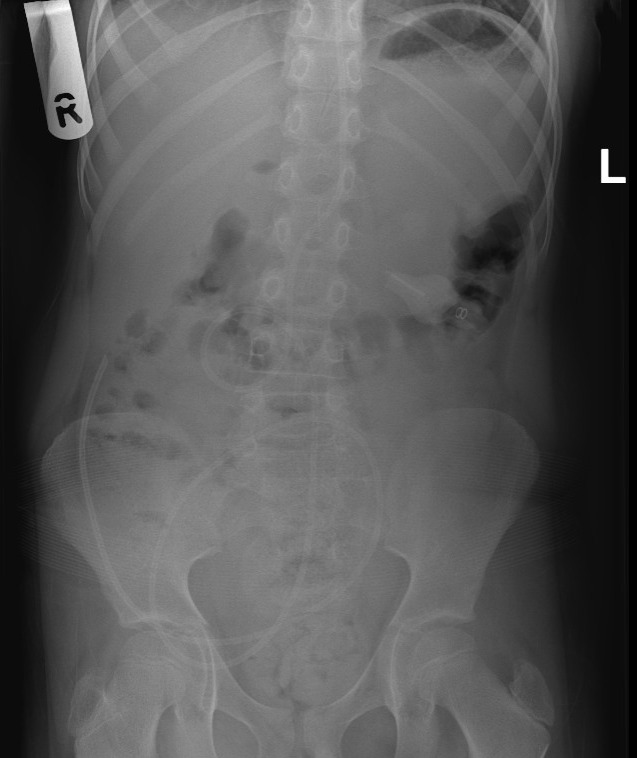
Abdominal X-ray demonstrating ventriculoperitoneal shunt and location of its tip.
